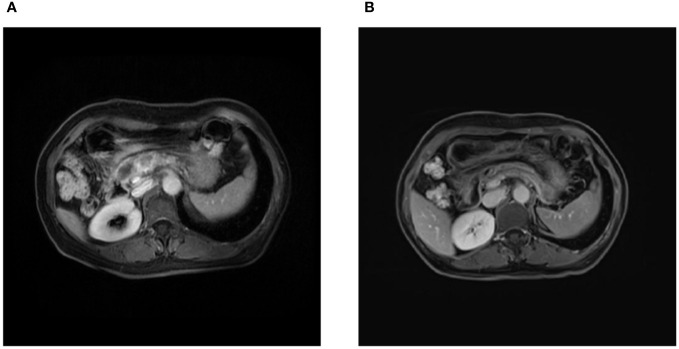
Magnetic resonance imaging findings. (A) Initial magnetic resonance imaging on admission revealed two masses in the neck and leptomeninges of the pancreas, respectively, along with an encapsulated exudate in the head of the pancreas. (B) Follow-up magnetic resonance imaging after 3 cycles of chemotherapy demonstrated a reduction in the size of the pancreatic masses.
